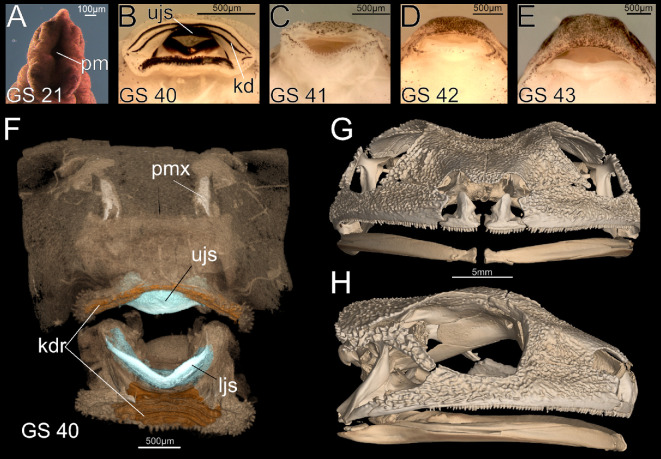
Anatomy and development of the feeding apparatus in the Cuban tree frog, *Osteopilus septentrionalis*. (A–E) Progressive development of the mouth: stomodeum (primary embryonic mouth; A), tadpole oral disc with keratinized mouthparts (B), atrophy of keratinized mouthparts (C), and formation of post-metamorphic frog jaws (D, E). (F–H) Micro-computed tomography scans of tadpole feeding apparatus (F) and adult skull with a toothed upper jaw and edentate mandible (G and H). Abbreviations: kd, keratodonts; kdr, keratodont ridges; ljs, lower jaw sheath; pm, primary mouth; pmx, premaxilla; ujs, upper jaw sheath. See the electronic supplementary material, Methods for scanning protocol.
